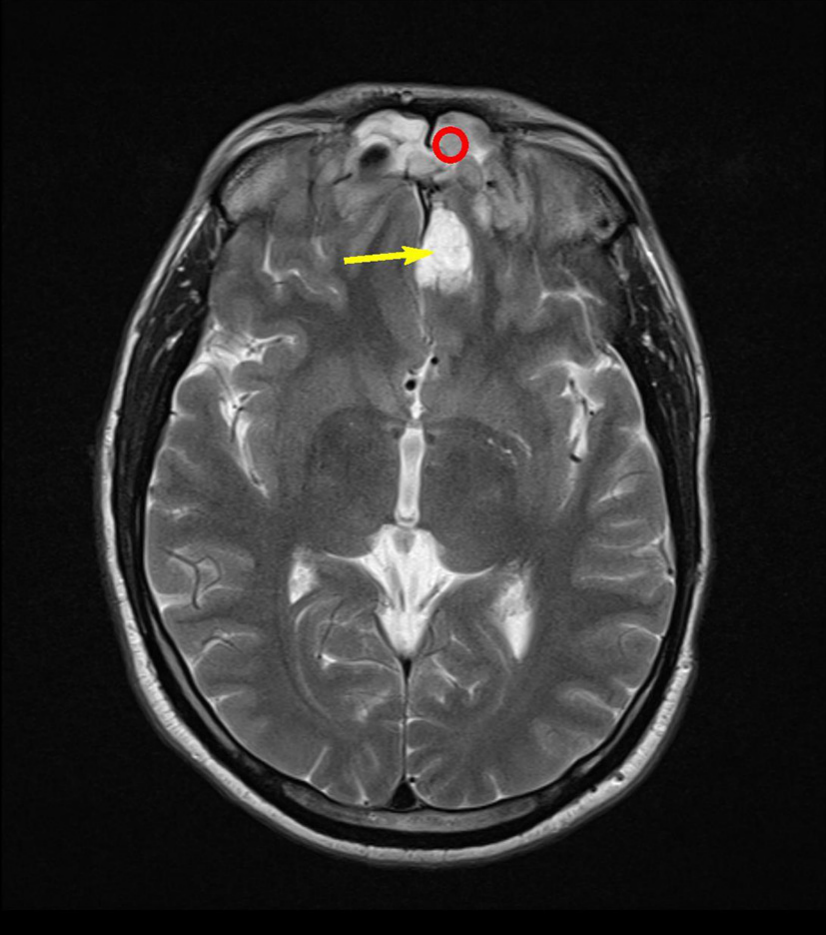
Axial *T*_2_ weighted MRI showing cranial extension of the mass (red circle) along the anterior falx into a cystic part (yellow arrow). No oedema is seen in the frontal lobe parenchyma.
